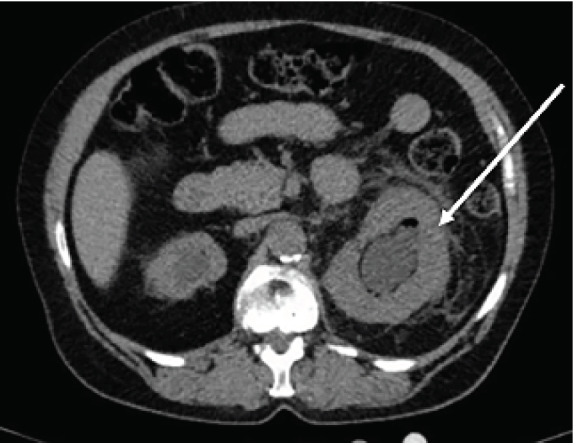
Computed tomography of the abdomen and pelvis showing an enlarged left kidney with hydronephrosis as well as air in the renal pelvis (white arrow).
